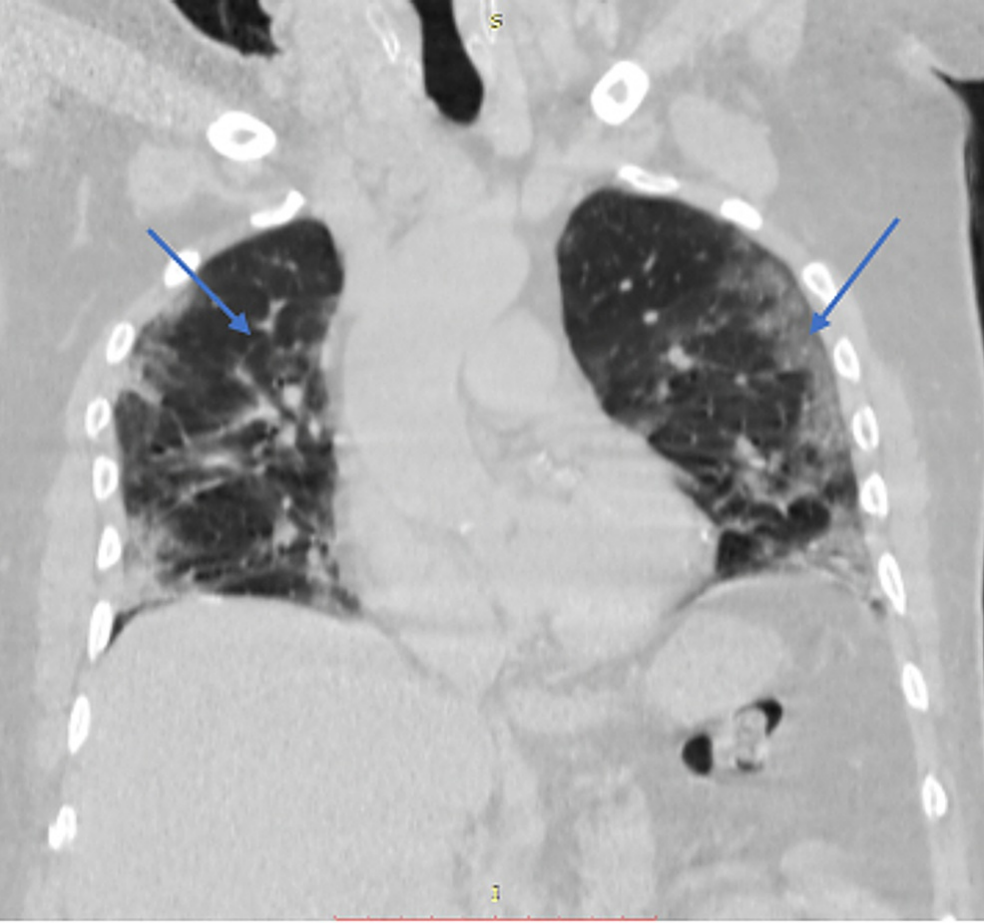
High-resolution CT (HRCT) scan of the chest with a coronal view of the patient with ground glass opacities bilaterally as shown by arrows with a CT severity score of 31/40
